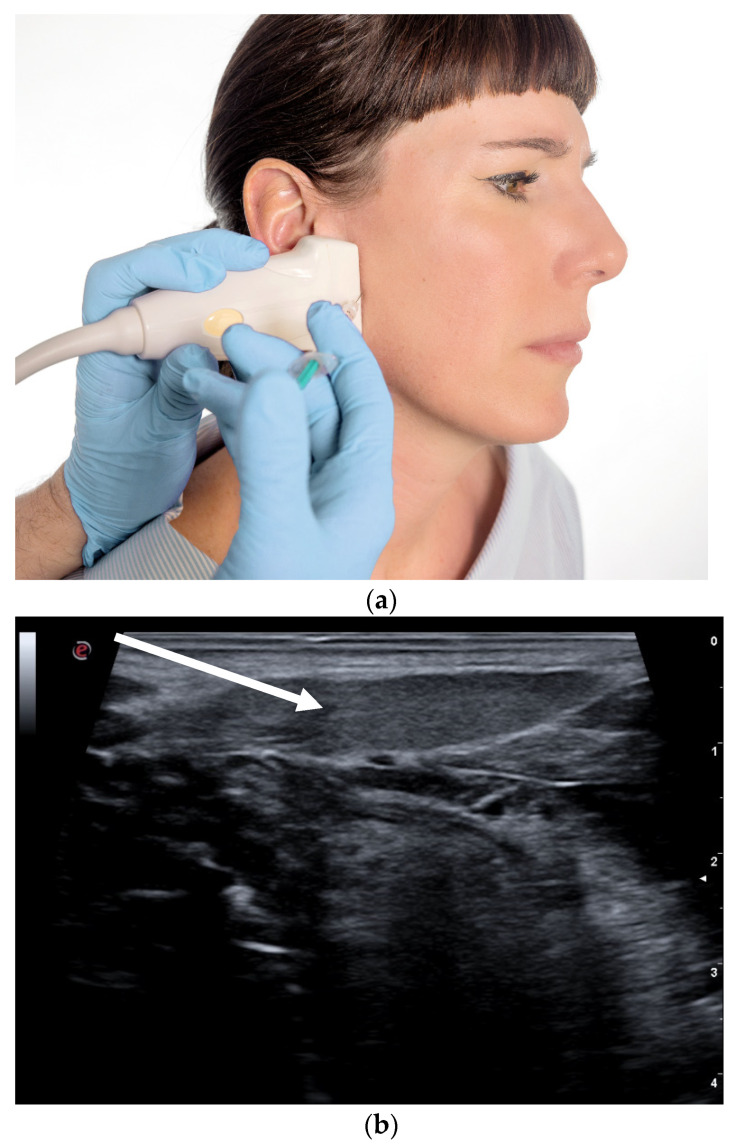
(a) Injection into the parotid gland; (b) sonography of submandibular gland.
